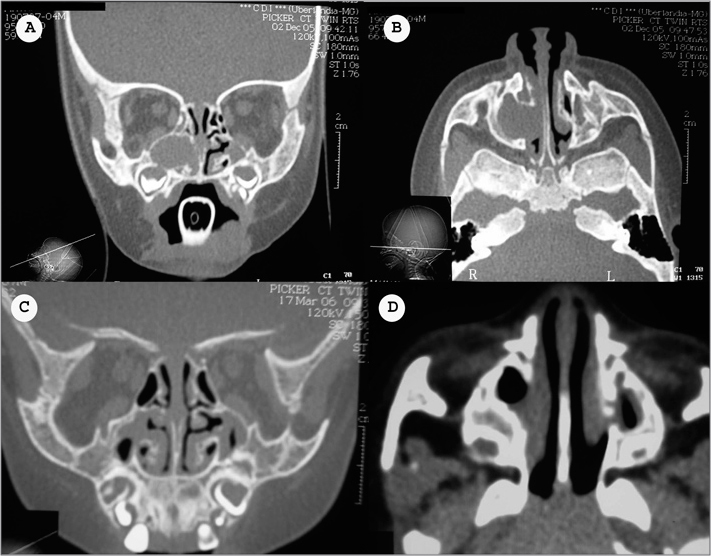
Computed tomography of the paranasal sinuses in axial and coronal sections showing a lesion with soft tissue density in the right maxillary sinus, bone erosion, and invasion of the ipsilateral nasal cavity (A and B). Eight months following surgery (C and D) there is edema of the mucosa in both maxillary sinuses, but no signs of recurrence of mucocele.
